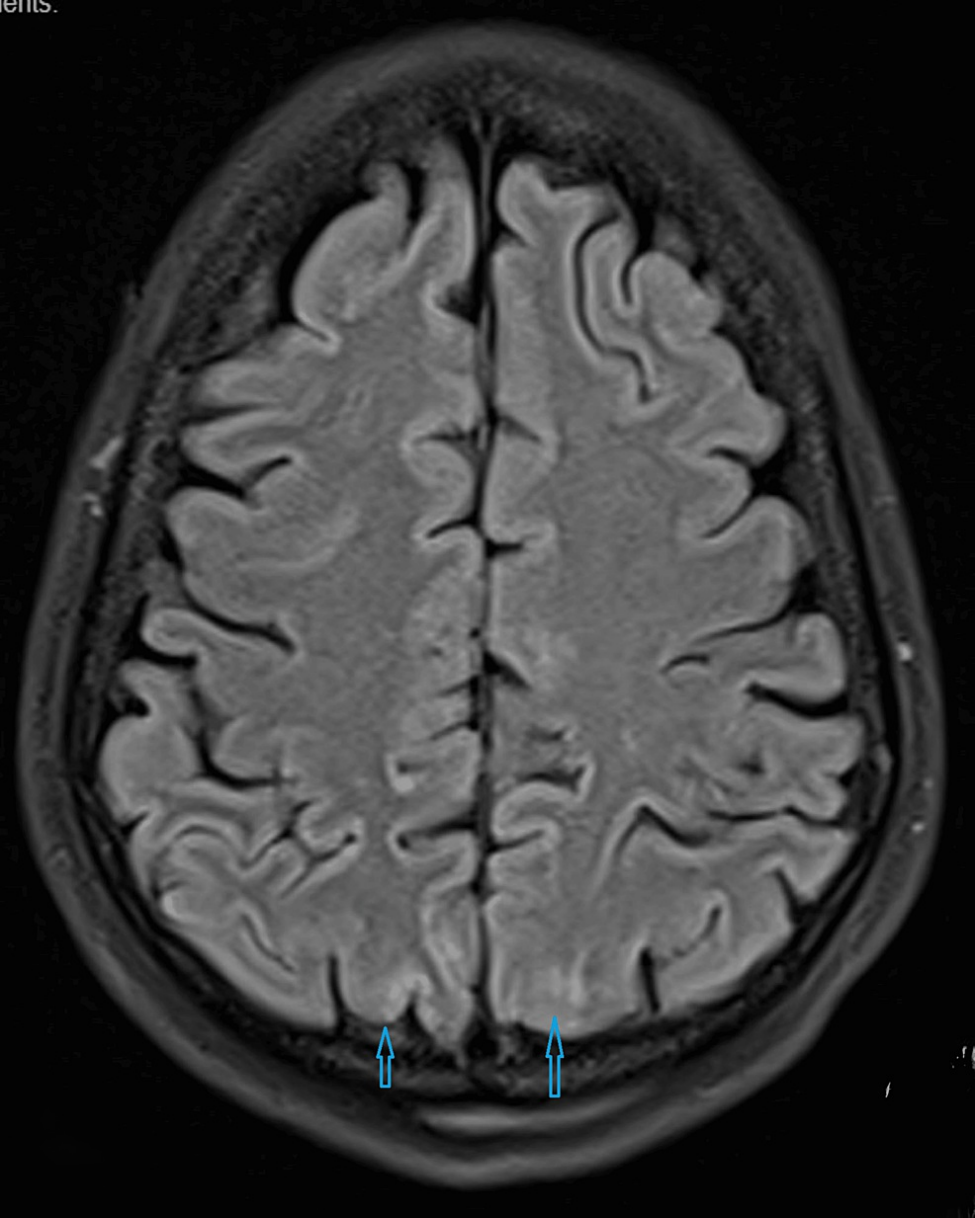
Magnetic resonance imaging (MRI) brain Subtle hyperintensity in bilateral parieto-occipital lobes in cortical and subcortical areas and bilateral cerebral hemispheres indicating posterior reversible encephalopathy syndrome (PRES)
